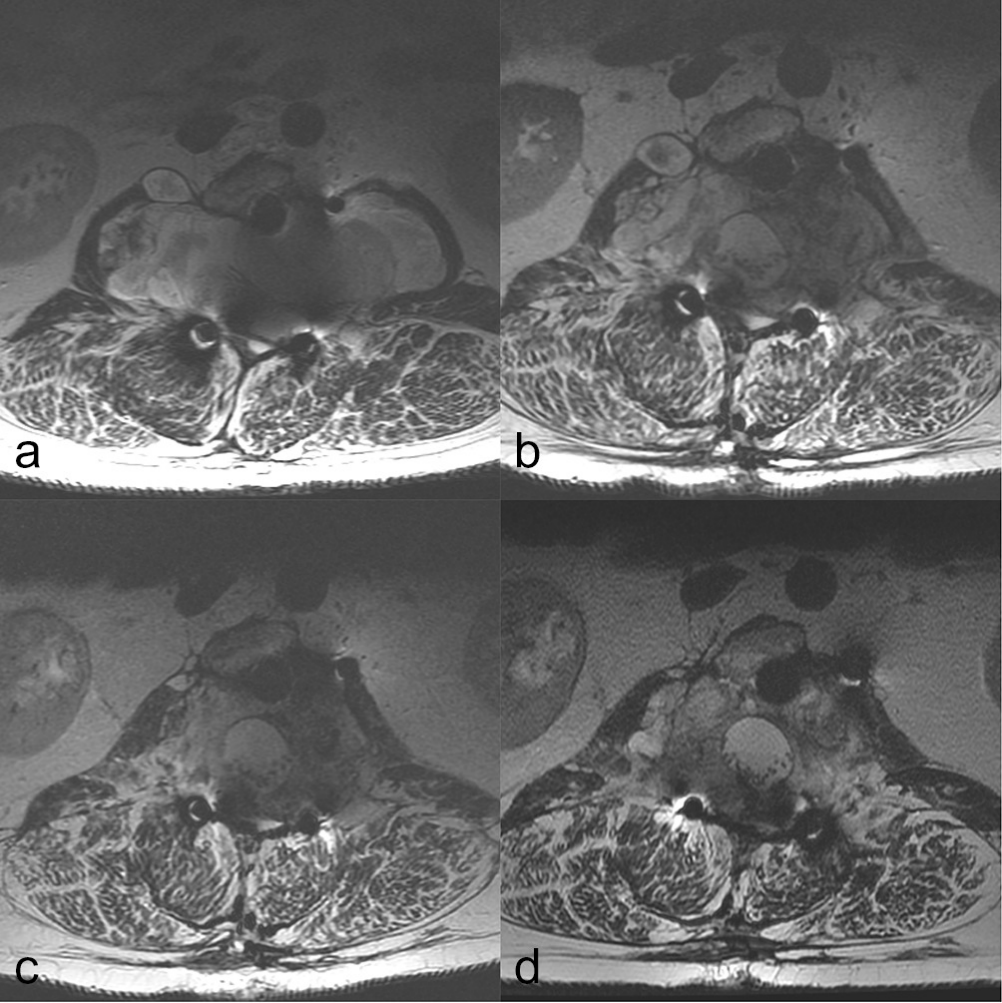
T*_2_ weighted MRI scans at the level of L2. Note the artefact caused by the metal reconstruction, which consists of a central vertebral cage and posterior rods. (a) Pre-radiotherapy (in 2010). A large mass is seen centred on the spine and involving the psoas muscles bilaterally. Tumour involves the body of the vertebra. A separate nodule is seen on the right psoas anteriorly. The whole spinal canal is filled with tumour and the nerve roots cannot be resolved separately. (b) Year 1 post-radiotherapy, (c) Year 3 post- radiotherapy, (d) Year 5 post-radiotherapy. Substantial reduction in the size of the mass, and the lumbar nerve roots can now be seen.
